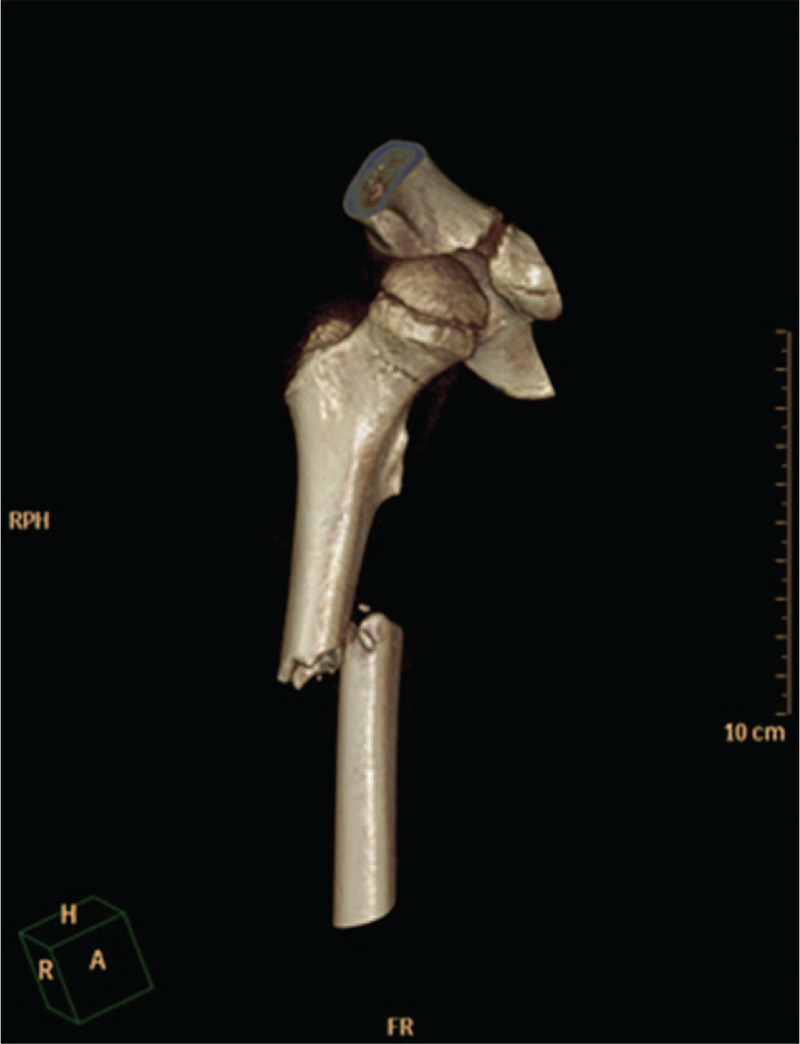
Preoperative three-dimensional CT examination of the right femur can clearly observe the femoral neck fracture line.
